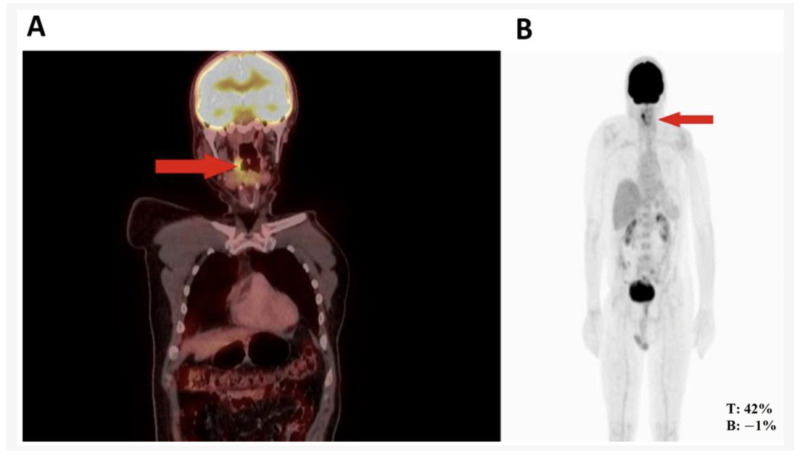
(A) Nodule in right pharynx and palatoglossal arch measuring 22 × 10 mm, SUV 6.5. (B) Hypermetabolic nodular thickening of right lateral pharyngeal wall, nonspecific, suspicious for neoplastic involvement. Taken from Montenegro et al. 2024 [13]. Used with permission.
